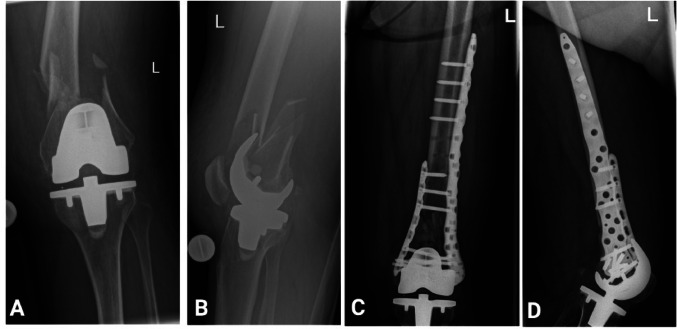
Case example of a Lewis–Rorabeck Type II periprosthetic distal femur fracture. Pre- and postoperative radiographs of a 72-year-old female patient with a Lewis–Rorabeck type II periprosthetic distal femur fracture sustained after a low-energy fall while gardening. A Preoperative anteroposterior and B lateral radiographs of the knee demonstrating the fracture. C Postoperative anteroposterior and D lateral radiographs following fixation with medial and lateral double plating, performed on the day of hospital admission
